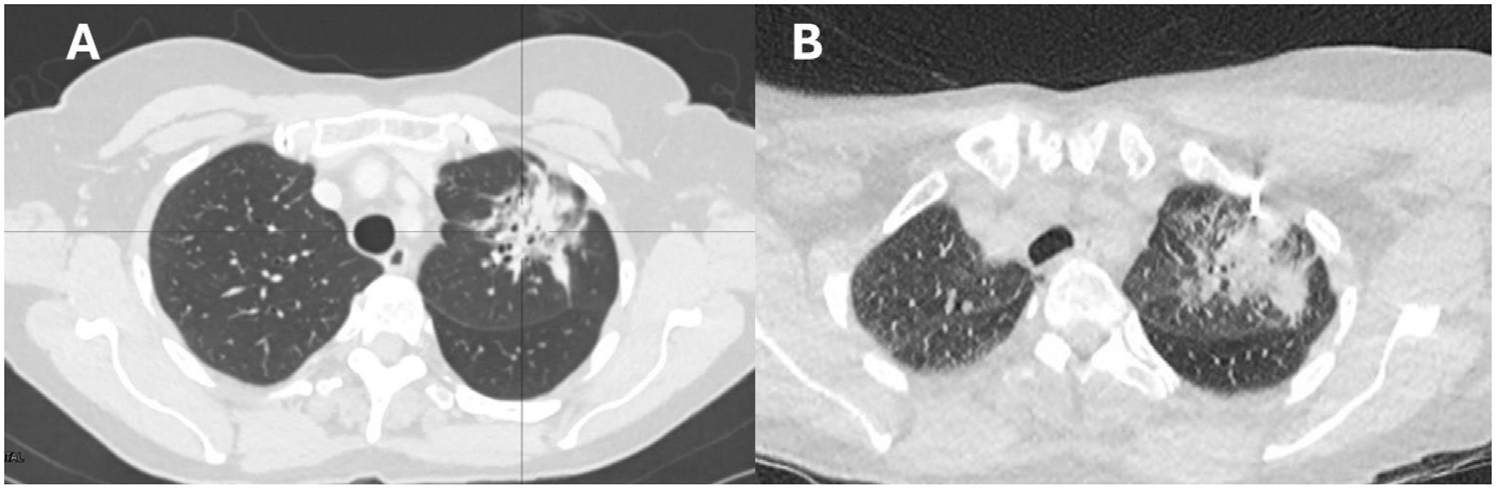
CT scan lung view (A) left upper lobe consolidation (B) biopsy needle.
